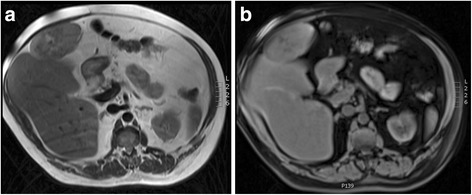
Abdominal MRI displaying the liver lesion. a T2WI. b T1WI
